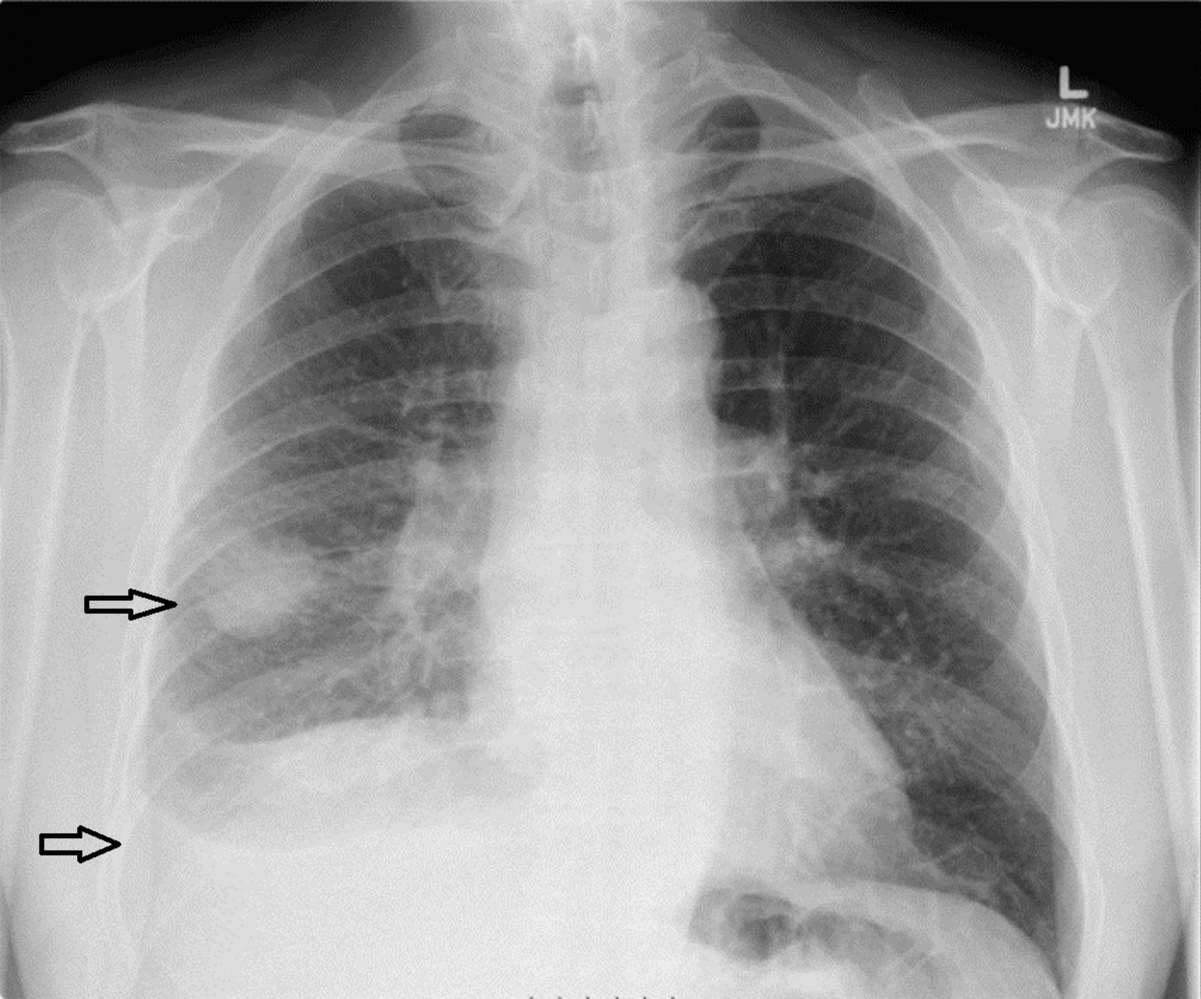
The chest X-ray revealed a 34 mm lesion in the right lung and small right-sided pleural effusion.
